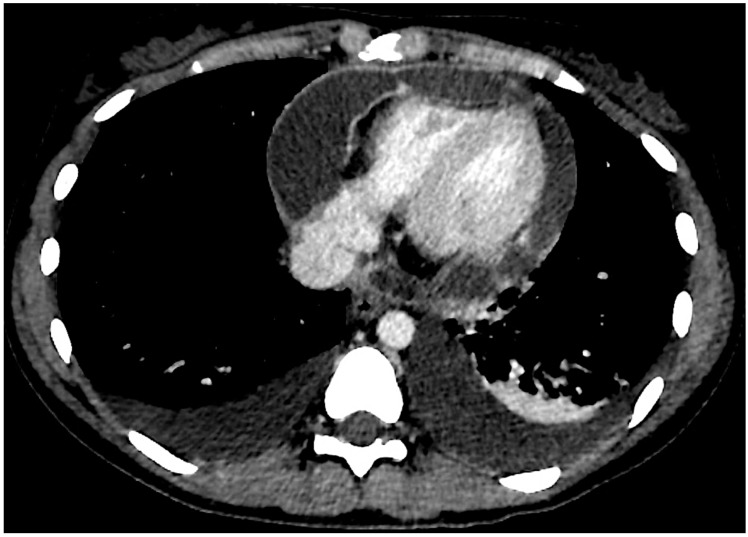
Axial chest CT scan section showing pleural and pericardial effusion. Pericardial enhancement is visible.
